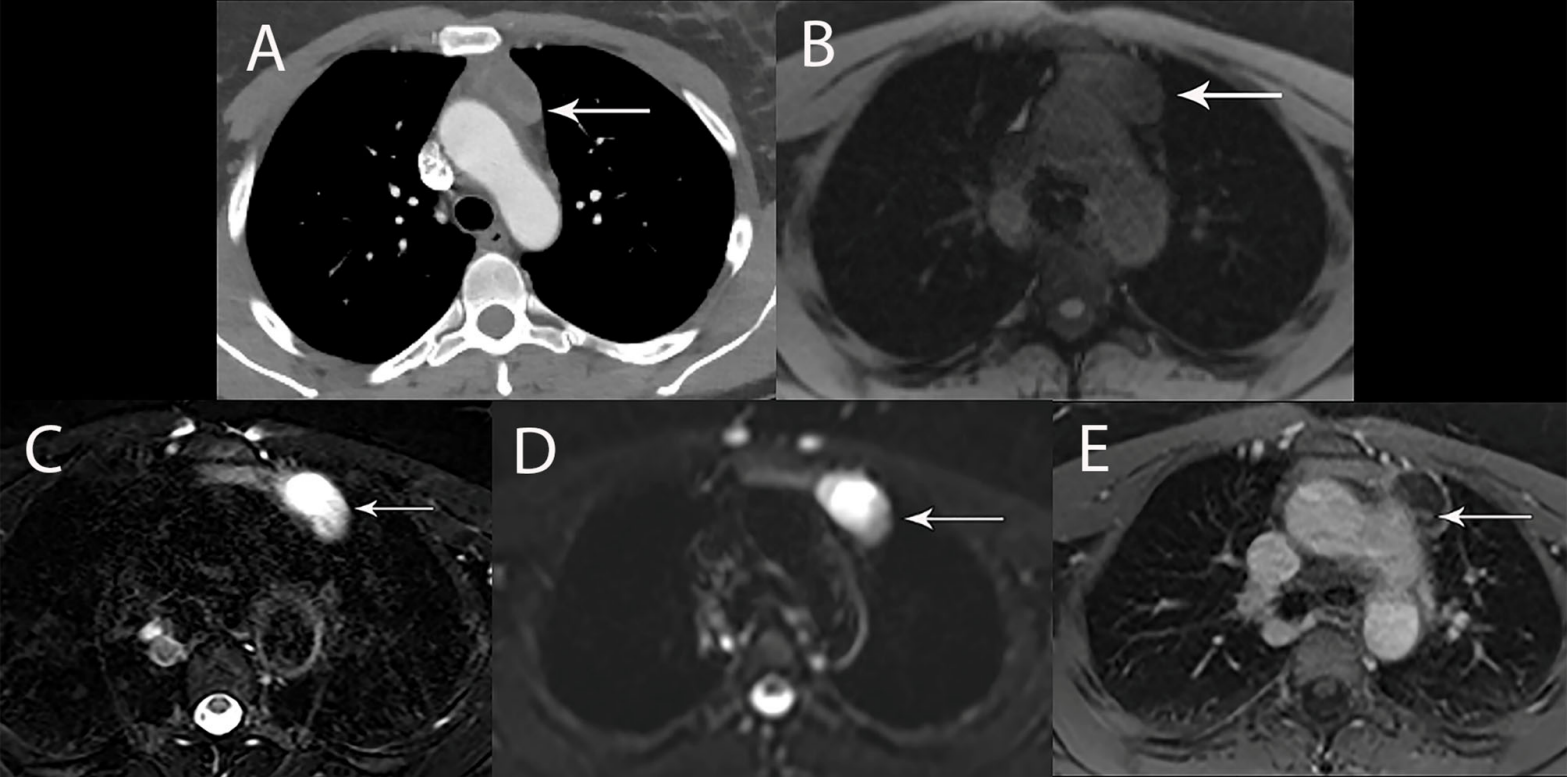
44 year old woman with cystic thymoma. (A) Contrast enhanced CT shows solid component along the posterior aspect. MRI showed intermediate signal intensity on T1 weighted (B), high signal intensity on T2 weighted images (C) and DWI (D) consistent with cystic component. The small solid component (arrow) shows enhancement on post contrast T1 weighted images (E).
